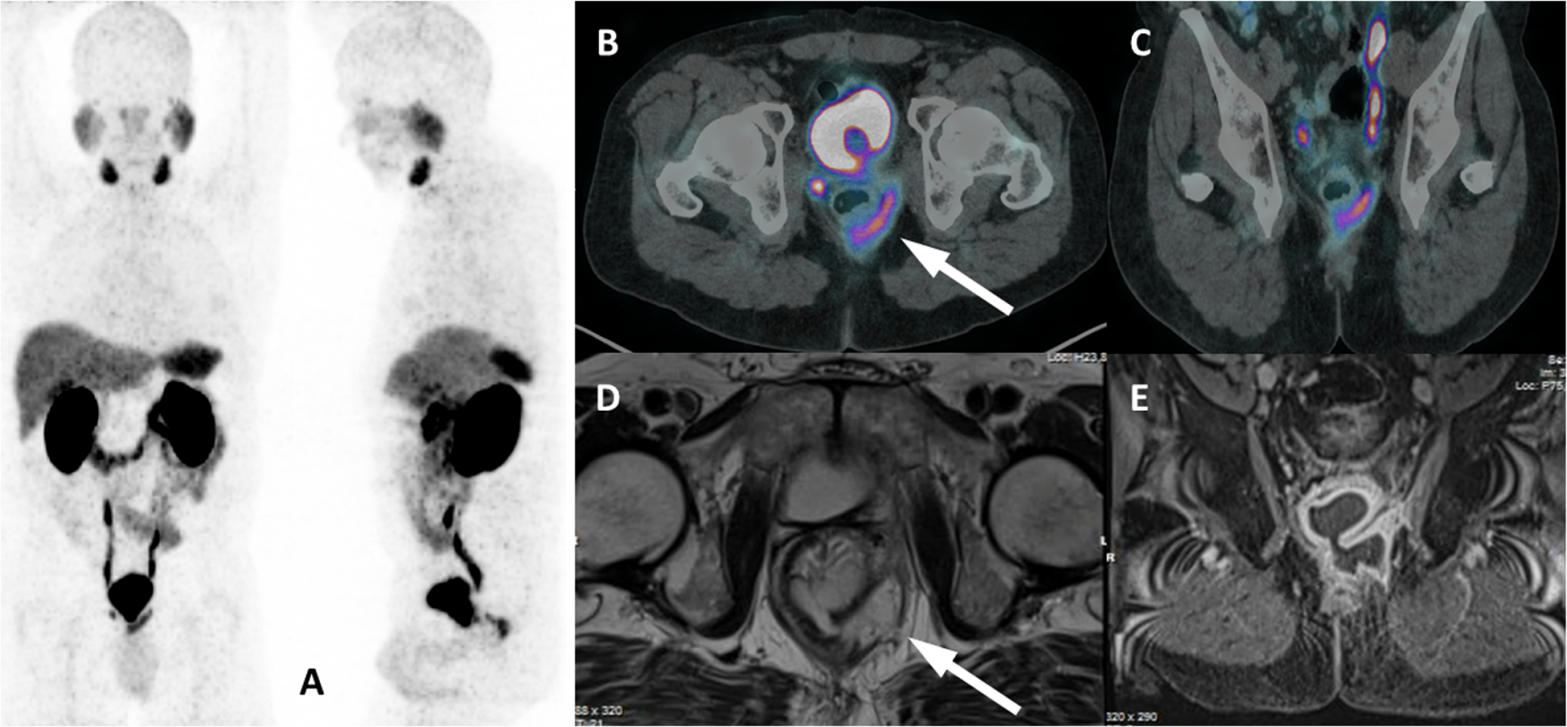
Perianal fistula with PSMA uptake. ^68^Ga-PSMA-PET MIP (a), axial PET/CT (b), coronal PET/CT (c) and axial T2w MR (d) and coronal T1w with gadolinum (e) show a perirectal fistula in the posterior left ischiorectal fossa (arrows)
